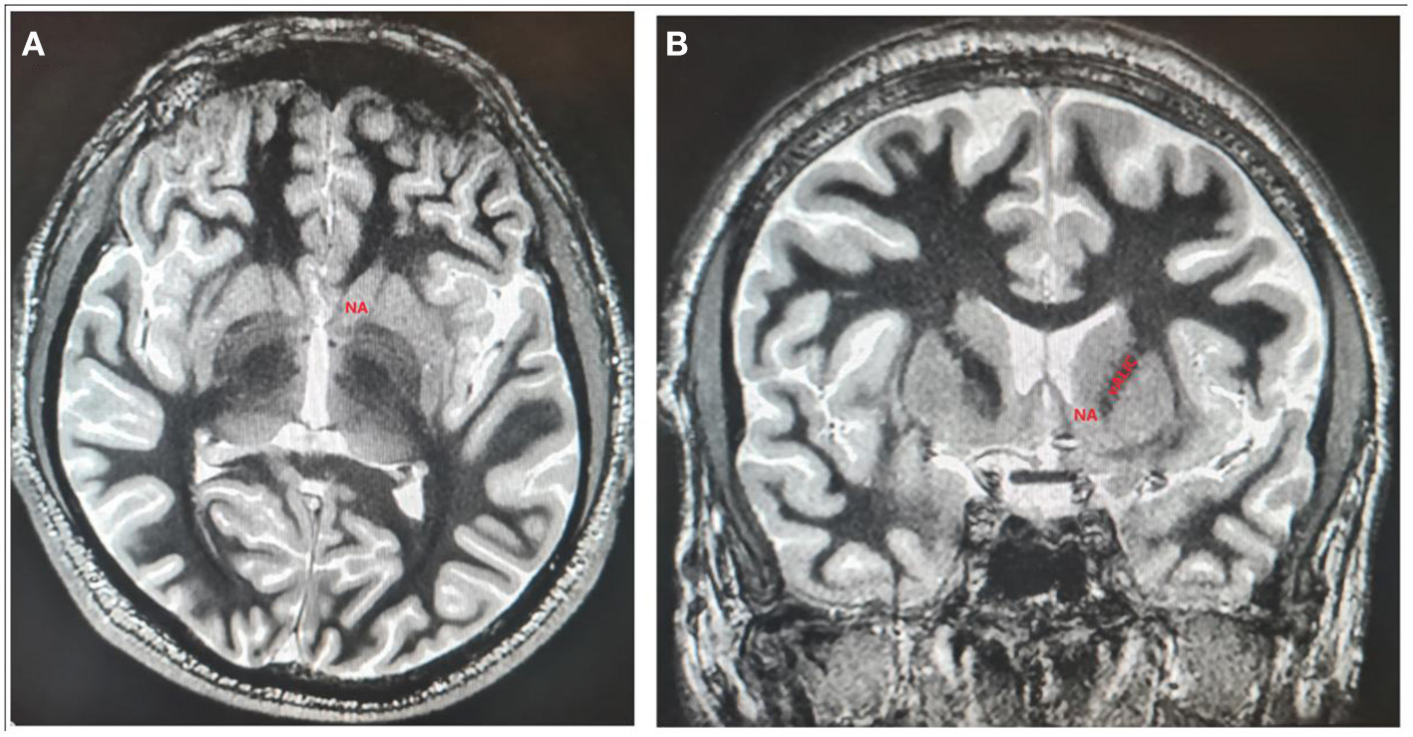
The localization of NAc and vALIC presented on axial (A) and coronal (B) sections of 3D T1-weighted imaging white/matter null sequence.
